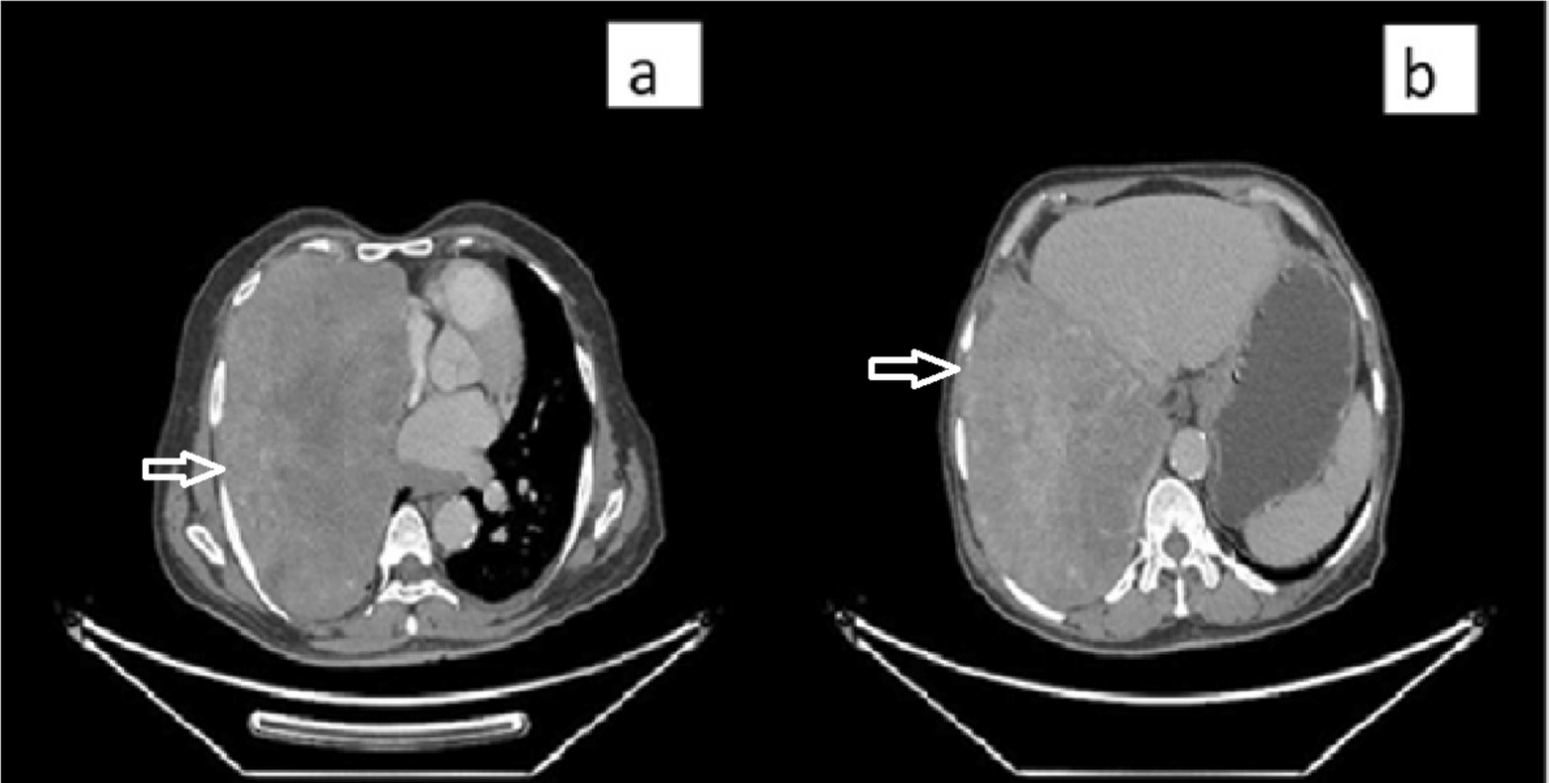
Computed tomography showing large well defined heterogeneously enhancing soft tissue mass lesions 20 × 13 x 19 cm with central scaring in the right hemithorax, causing complete collapse consolidation of the right middle lobe and partial collapse consolidation of the right middle lobe (a) huge heterogeneous mass pointing towards the heart not invading and are well capsulated (b) huge heterogeneous mass pointing towards the liver not invading and are well capsulated.
